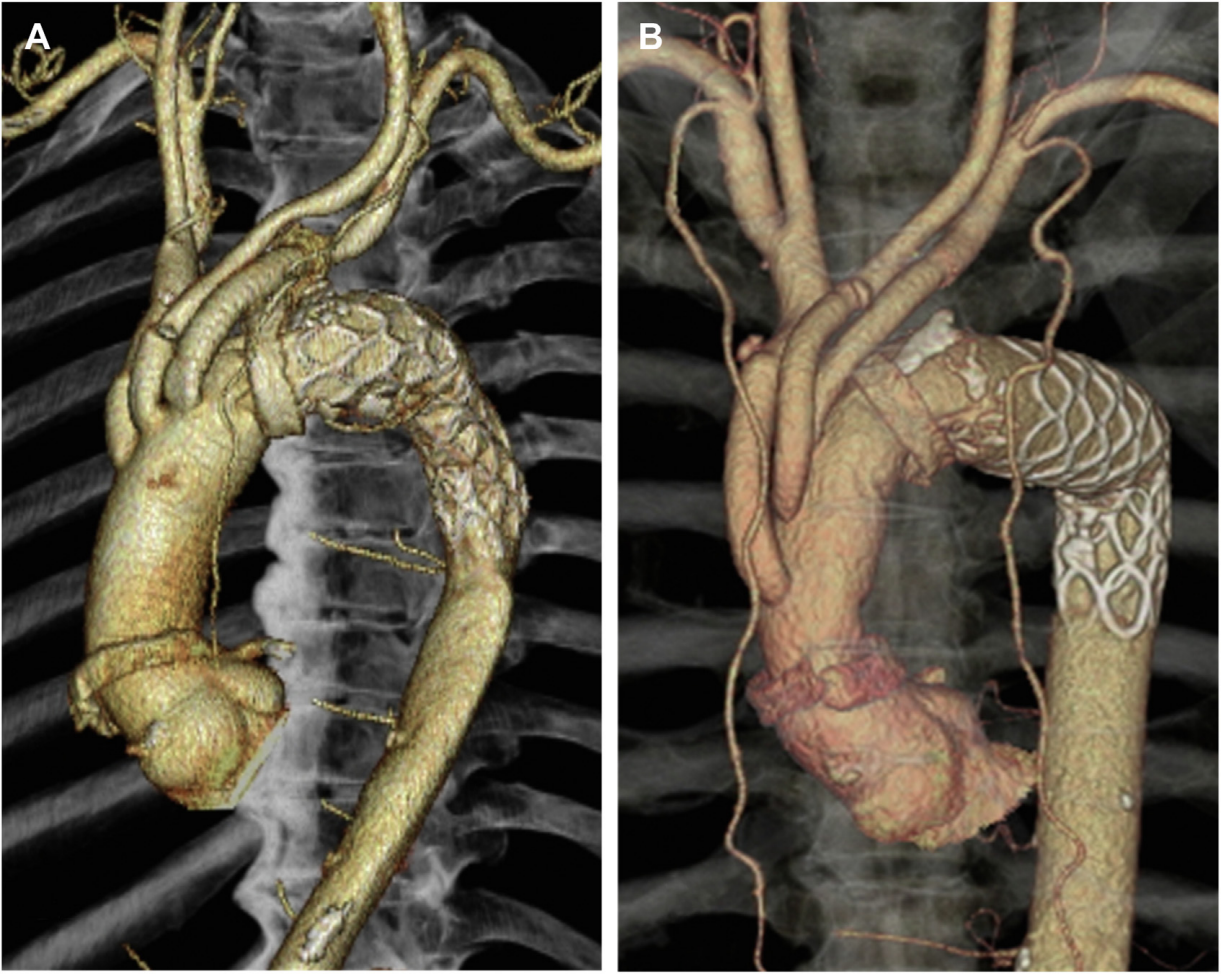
Postoperative 3-dimensional computed tomography images. (A) Patient 1 and (B) patient 2.
